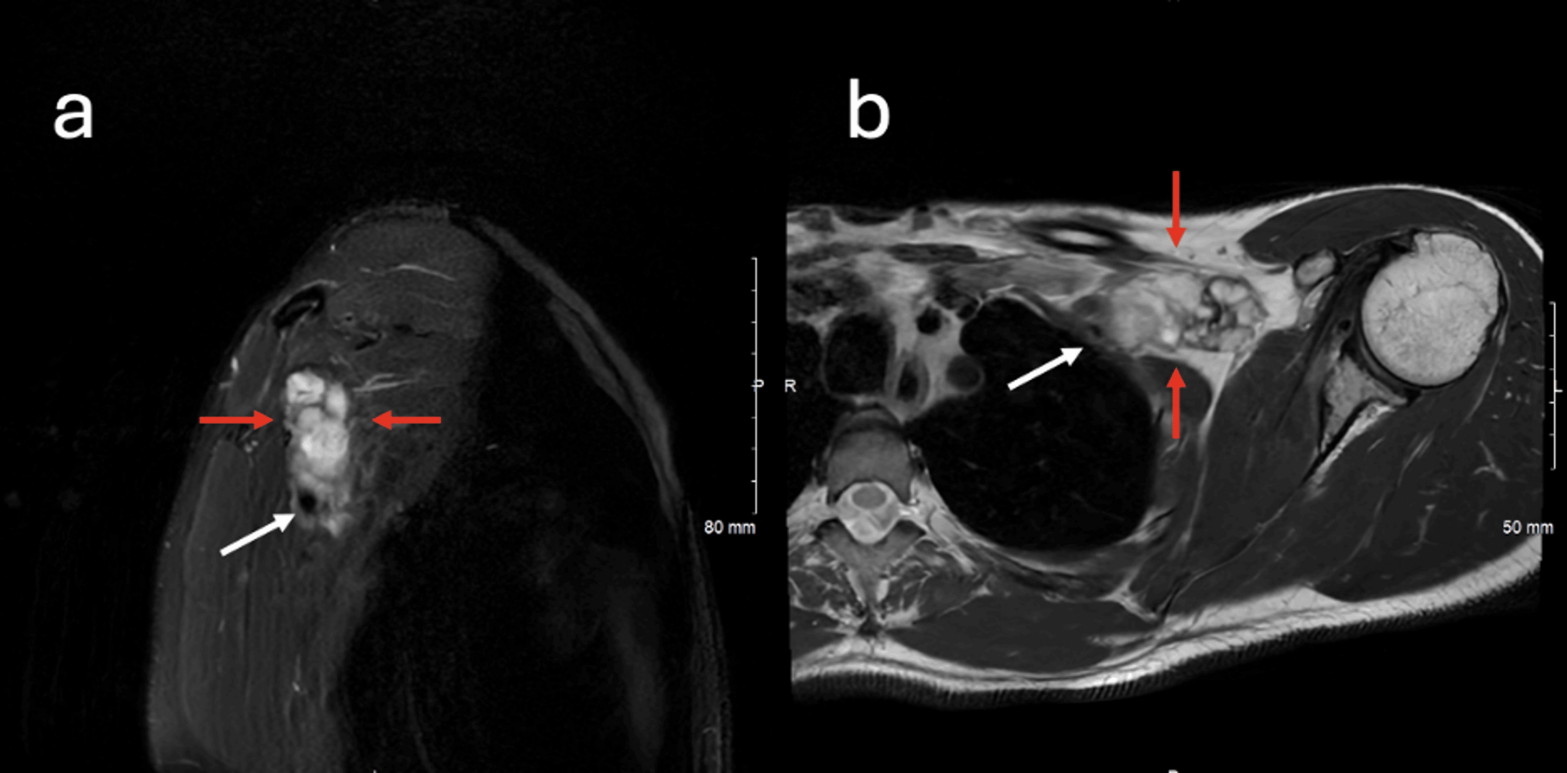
Sagittal and axial T2 MRI of the left brachial plexus demonstrating tumor relationship to axillary and brachial arteries Sagittal T2 fat-saturated image of the left brachial plexus (a) demonstrates the relationship of the left axillary artery (white arrow) with the adjacent tumor (red arrow). Axial T2 image of the left brachial plexus (b) demonstrates the relationship between the left brachial artery (white arrow) and the cystic tumor (red arrows). Note that for purposes of surgical planning, the lesion is cephalad and anterior to the left brachial artery.
